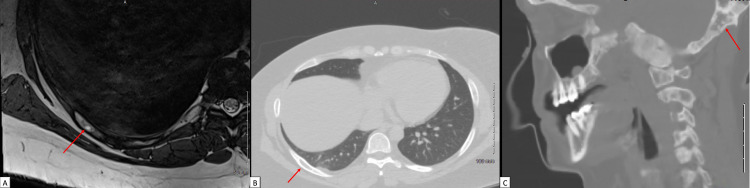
A 41-year-old, asymptomatic woman with Mazabraud’s and McCune Albright syndromes with an incidental intercostal intramuscular myxoma. Cross-sectional imaging demonstrating polyostotic fibrous dysplasia (A) Axial T2-weighted MR image of the chest shows hyperintensity and expansion (arrow) within the eighth posterior right rib. (B) The follow-up axial image from CT of the chest shows a well-defined expansile, cystic lesion (arrow) within the eighth posterior right rib. (C) The sagittal image from CT of the neck shows multifocal sclerotic and cystic changes notably involving the occipital bone (arrow), consistent with the patient’s known history of polyostotic fibrous dysplasia.
